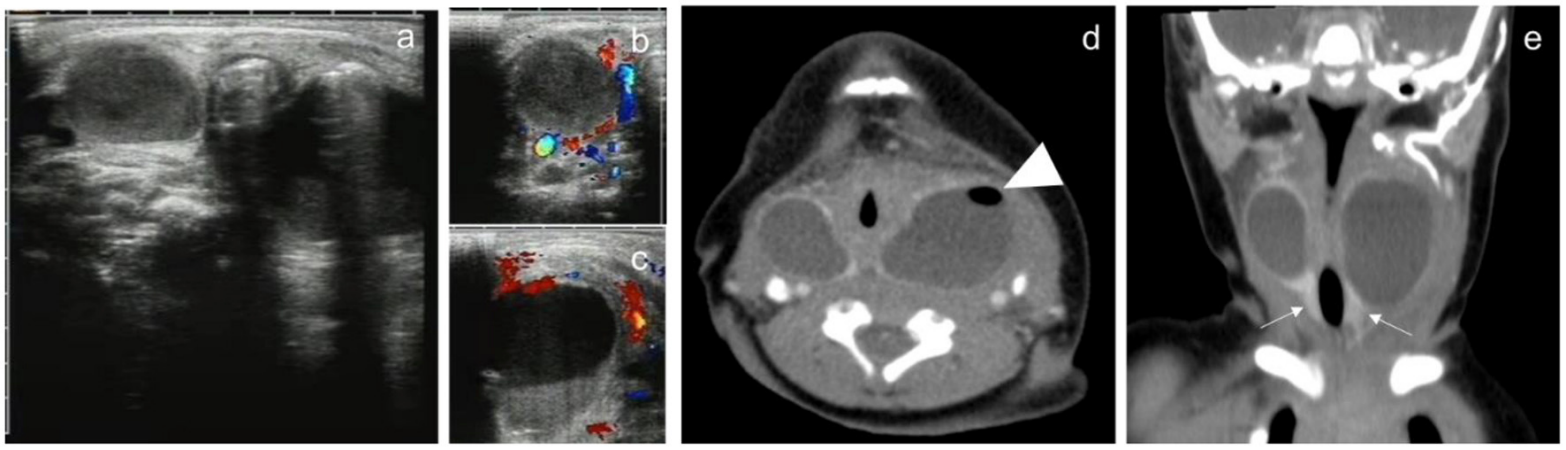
A 3-day male newborn with bilateral neck CPSF. Both US and contrast-enhanced CT showed bilateral cervical cystic masses with no septum. US presented inhomogeneous cystic hypoechoic with air-bubble containing (a). CDFI: there was no blood flow in the lesions (b,c). Contrast-enhanced CT showed the mass in the bilateral cervical visceral space penetrated into the retropharyngeal space, the wall of the cyst was thick and high attenuation, the border was well defined, and small bubbles could be seen in the cystic mass of the left neck (d, arrowhead). Coronal images showed bilateral cervical lesions involved the thyroid gland, and the thyroid enhancement density was homogeneous (e, arrow).
